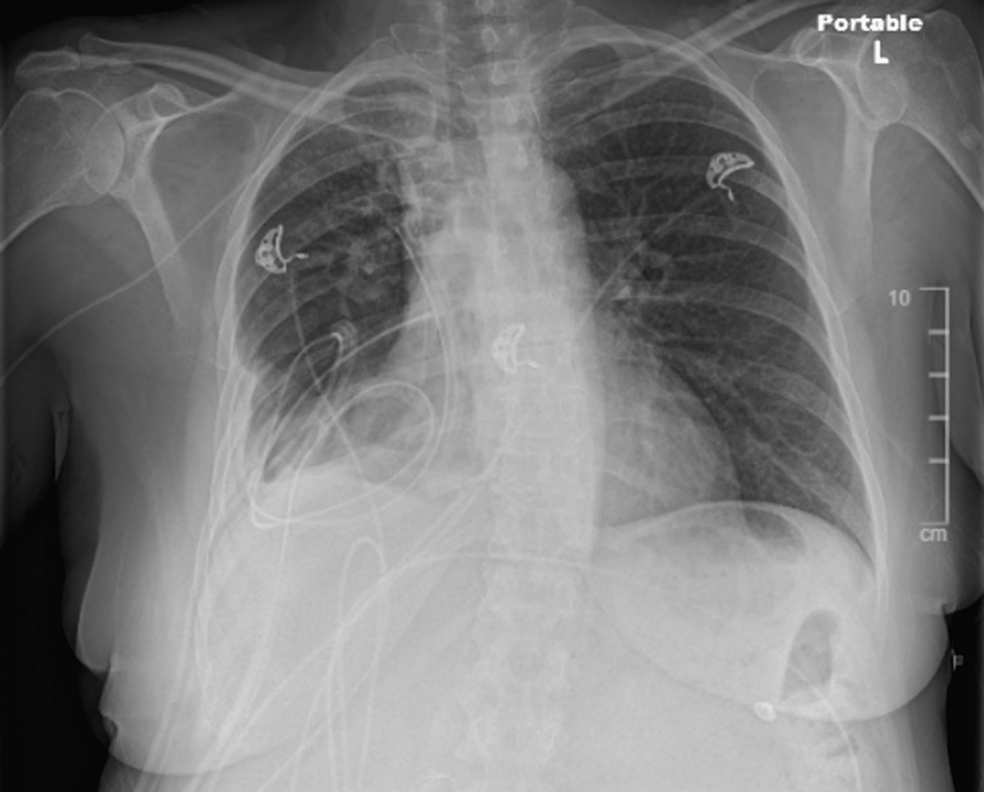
Chest X-ray Status Post-VATS, Postoperative Day 6 VATS: video-assisted thoracoscopic surgery
